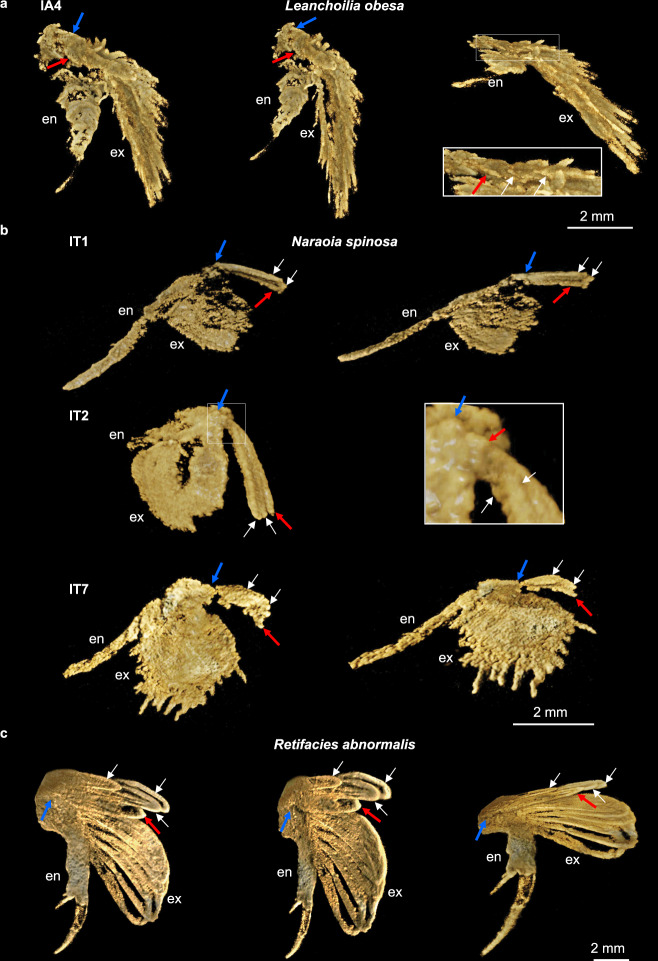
Computed tomographic images showing exite-bearing appendages. a
